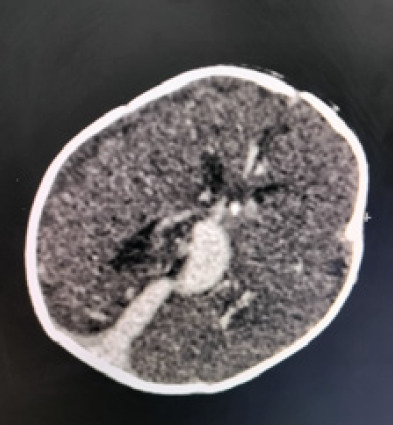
Contrast enhanced CT of head showing vein of Galen aneurysmal dilations with multiple dilated feeding arteries.
